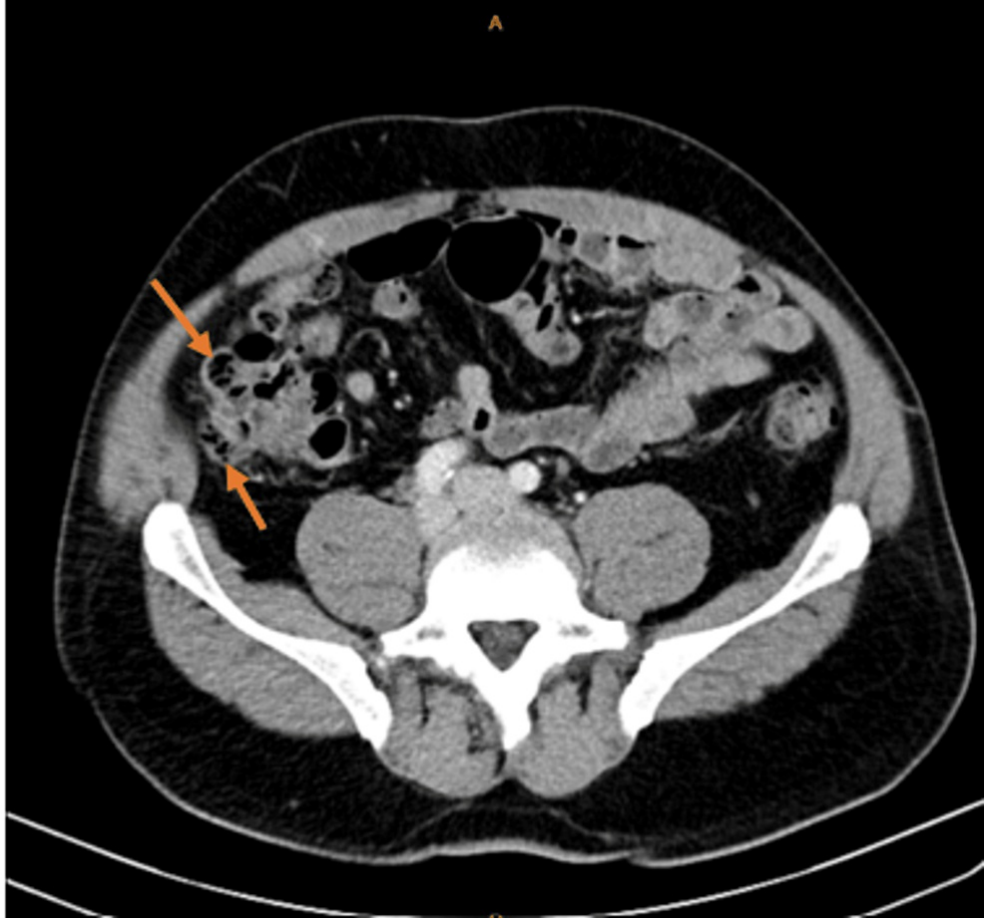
CT axial scan demonstrating right-sided diverticulitis.
